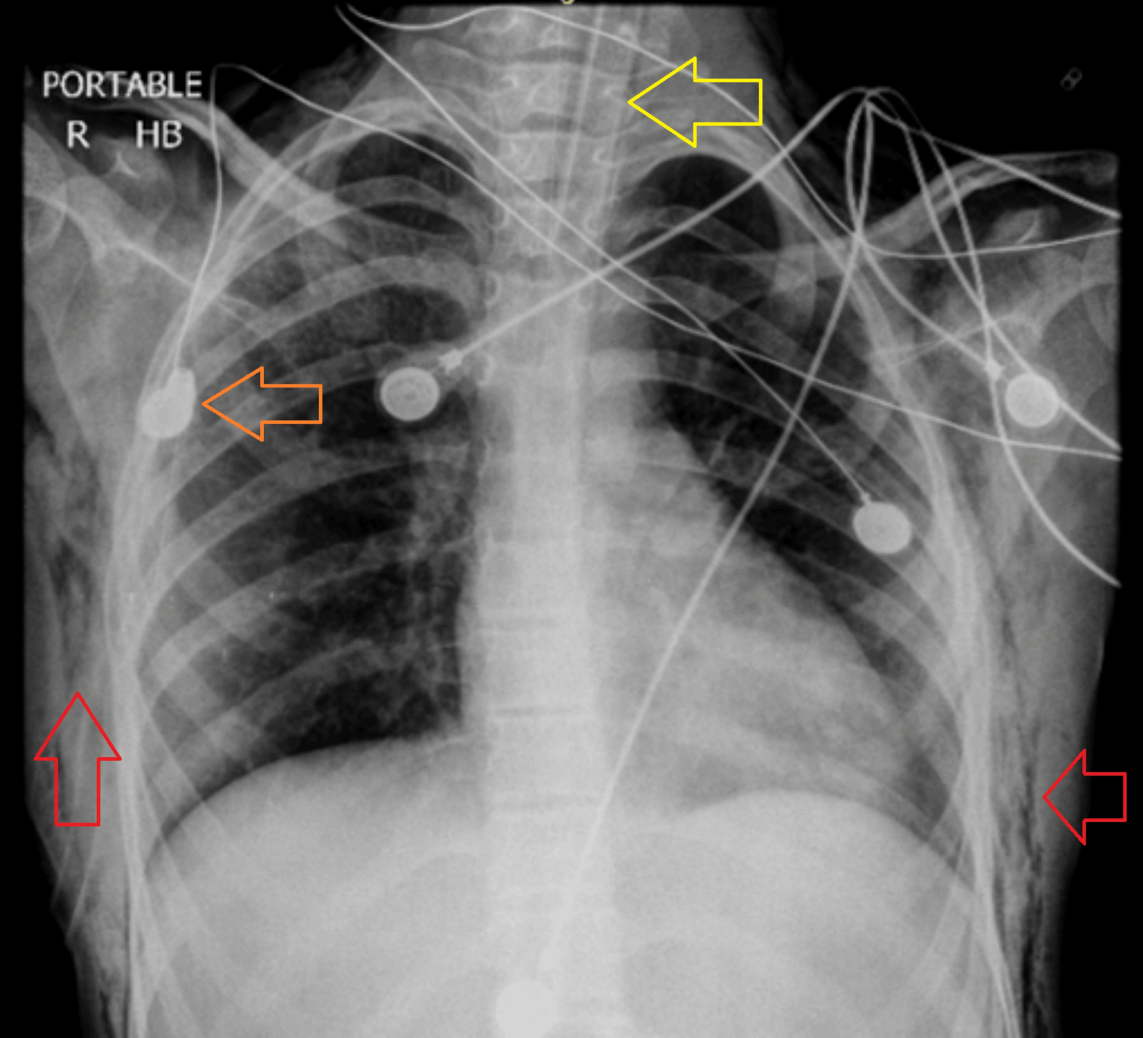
Chest X-ray taken on the day after passing the patient's chest tube (orange arrow) with the endotracheal tube in place (yellow arrow) and bilateral subcutaneous emphysema (red arrow)
